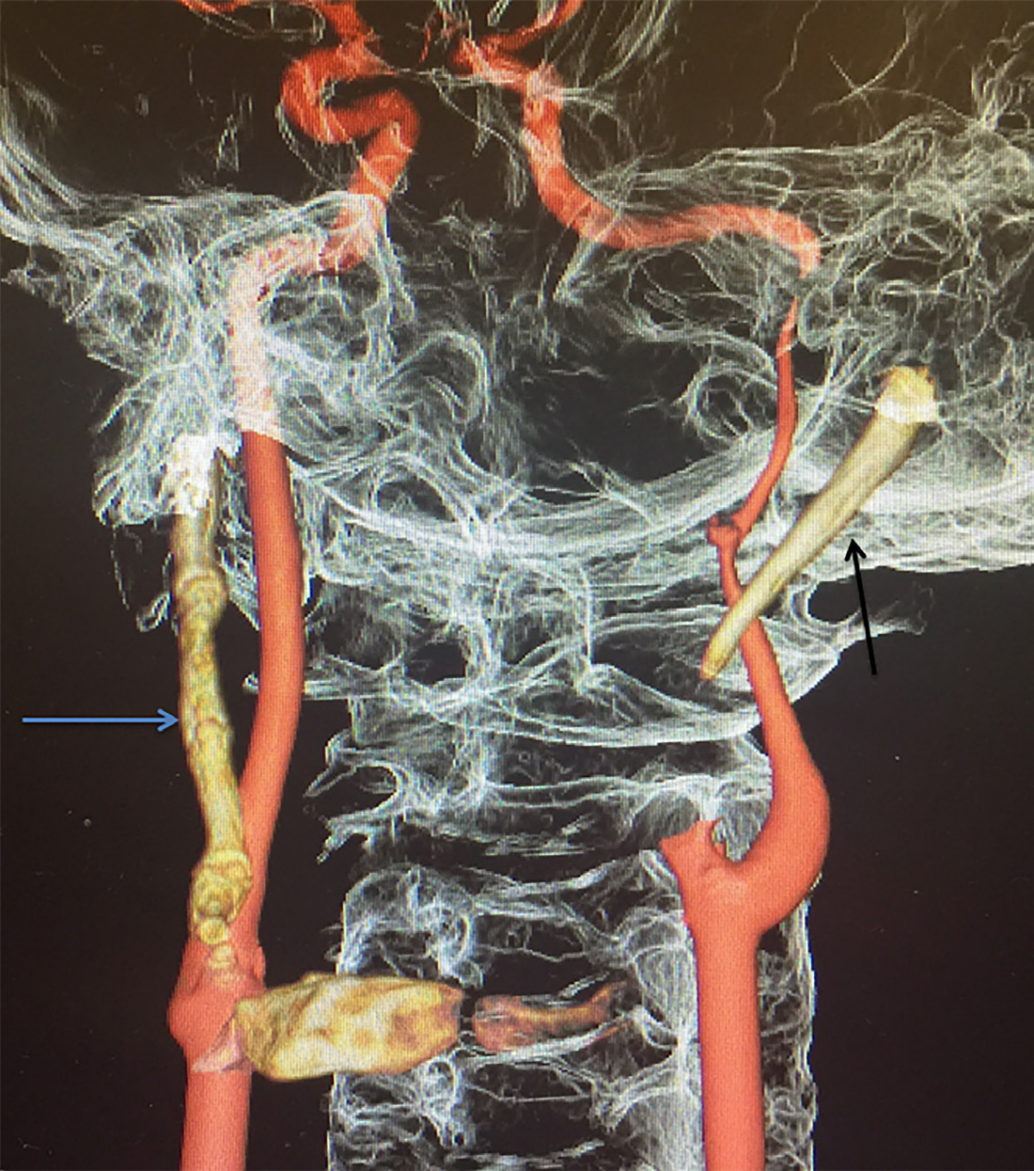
Computed tomography angiography with three-dimensional rendering showing large left styloid process (black arrow) and large completely calcified right stylohyoid ligament (blue arrow).
